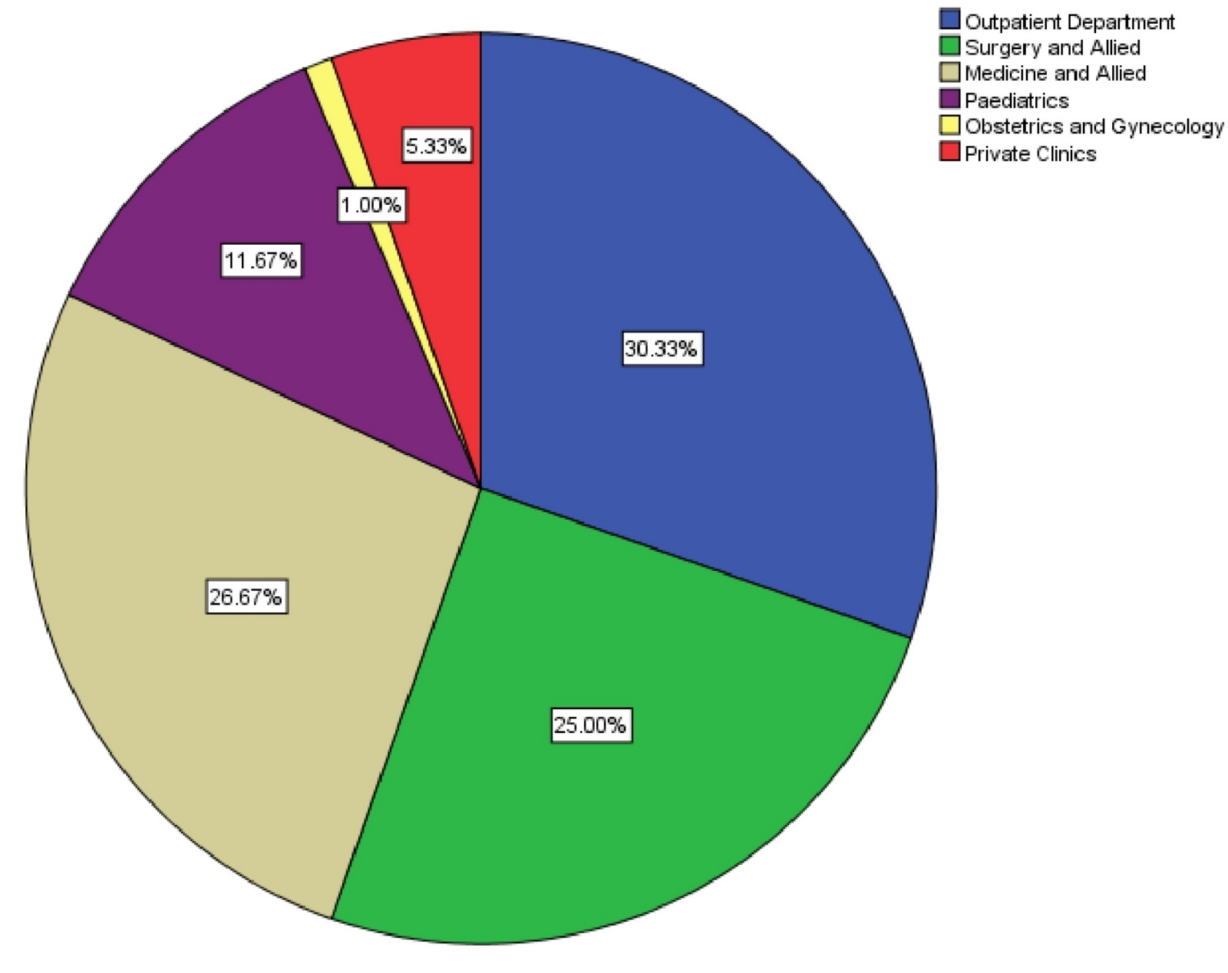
Source of the computed tomography (CT) scan request forms
